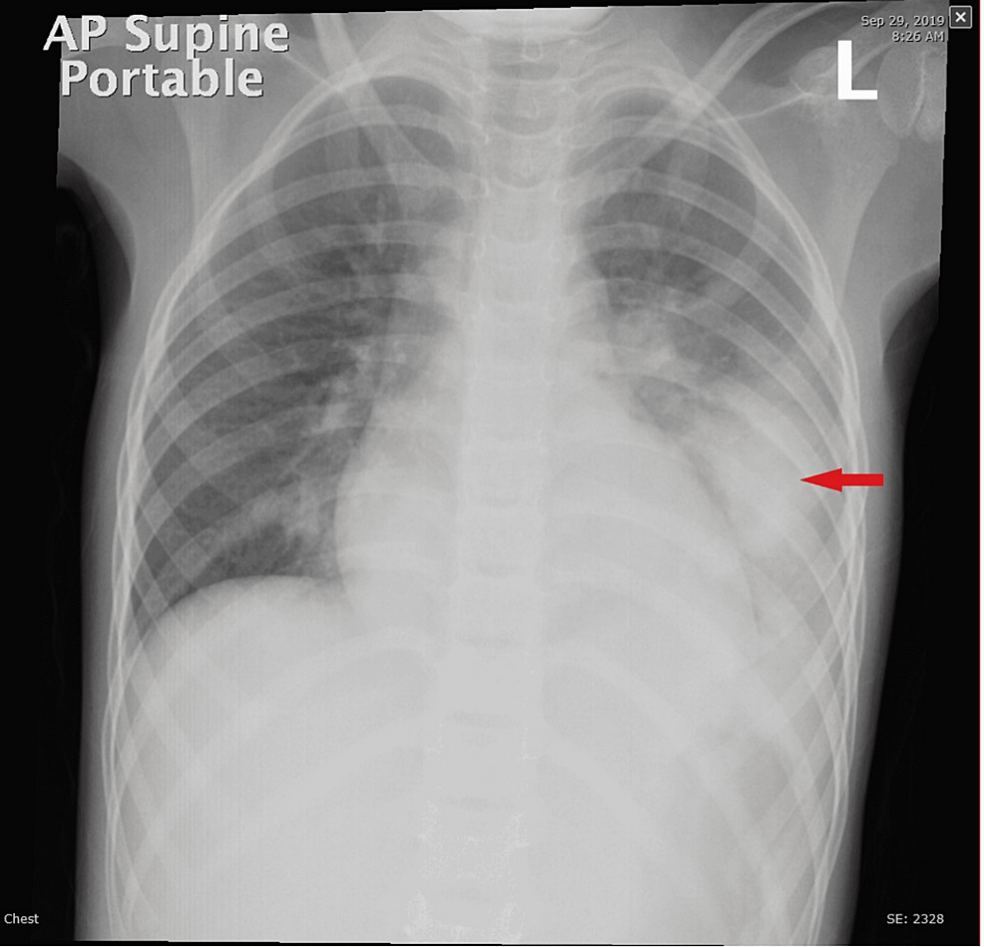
Chest X-ray Anteroposterior supine portable chest X-ray shows left-sided pneumonia (red arrow)
